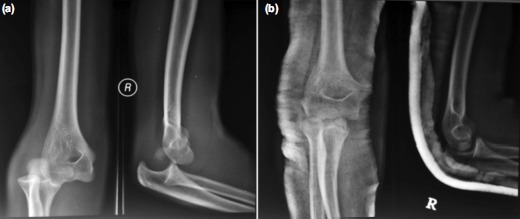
(a) Right elbow radiograph showing right posterolateral elbow dislocation with intraarticular entrapment of medial humerus epicondyle avulsion fracture fragment in the elbow joint. (b) Medial humerus epicondyle fracture fragment remained entrapped in the elbow joint after closed manipulative reduction.
